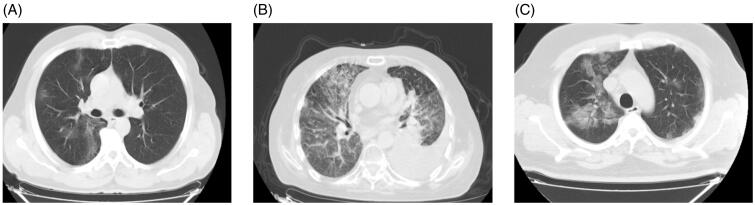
Chest CT images in COVID-19 patients at different stage of the disease (panels A–C). (A) An axial CT image obtained without intravenous contrast in a 62-year-old male shows bilateral peripheral focal ground-glass opacities (GGOs) with a rounded morphology; (B) an axial CT image obtained without intravenous contrast in a 59-year-old female shows consolidative opacities and pleural effusion on the left and ground-glass opacities including interstitial thickening on the right side; (C) an axial CT image obtained without intravenous contrast in a 29-year-old male patient demonstrates bilateral mixed ground-glass opacities and central consolidated areas of the lung.
